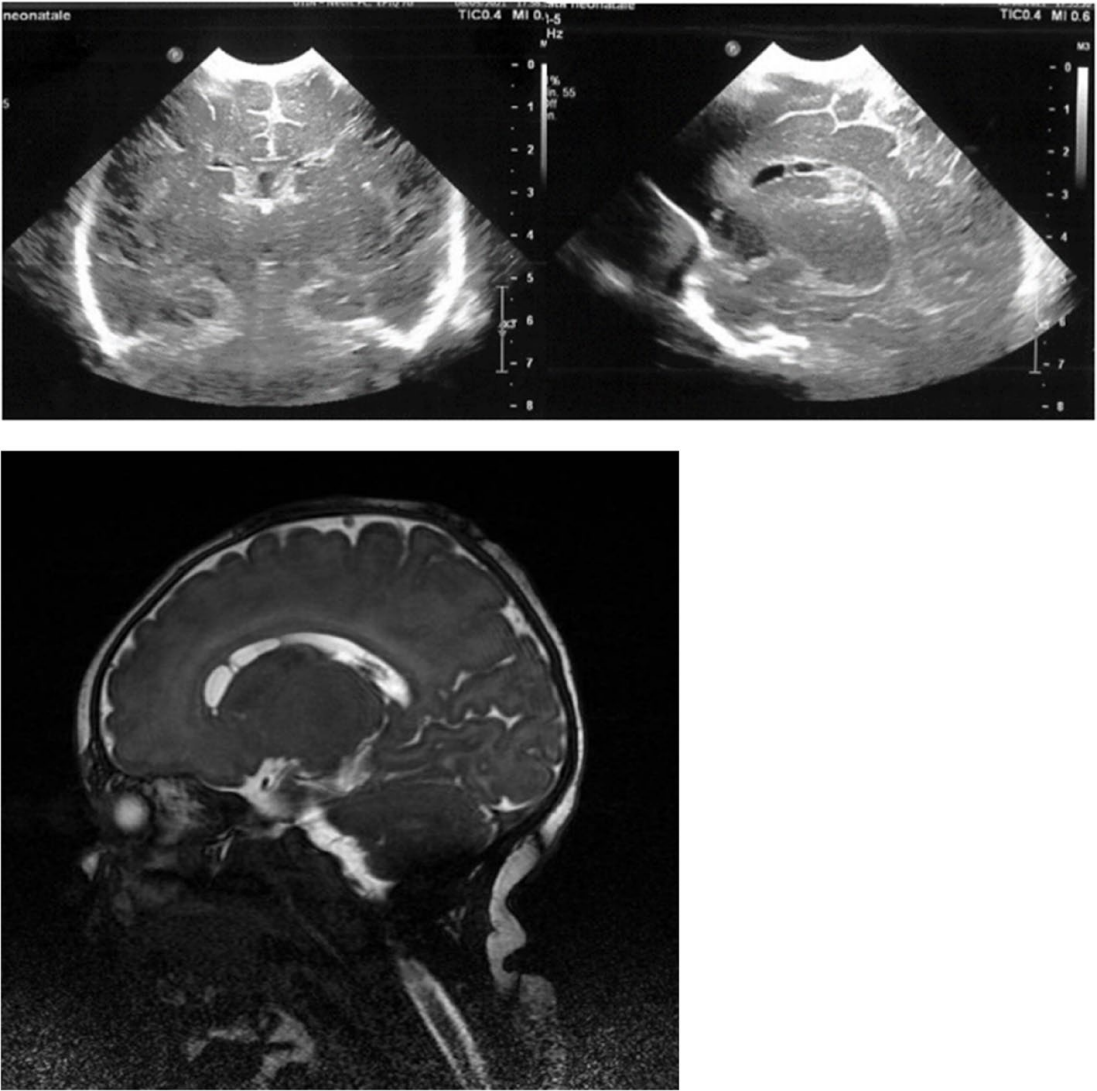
cUS and brain MRI of a term female newborn exposed to SARS-CoV-2 in utero at 22 W showing bilateral FHCs and bilateral subependymal cysts involving the caudate nucleus
